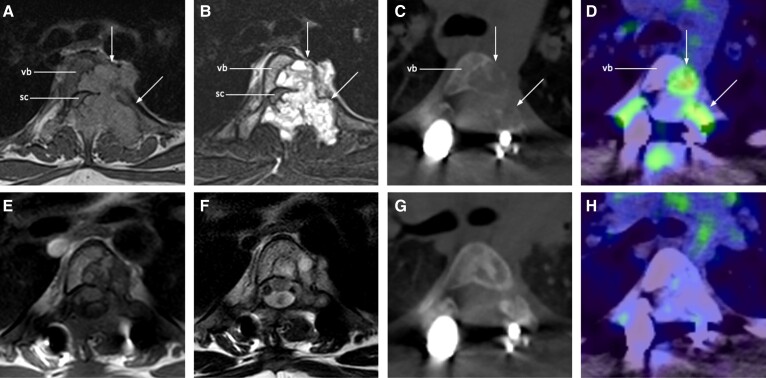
Representative images of denosumab treatment in spinal ABC. MRI T1-weighted (A) and STIR (B) images show a lobulated, expansile lesion (arrows) of T4 vertebral body (vb) and posterior elements with spinal cord (sc) stenosis, before partial resection and spinal stabilization with rods and bone graft. Postsurgery but before denosumab, the remaining ABC is seen as a lytic lesion in the left T4 vertebral body and pedicle on low-dose CT (C) with FDG avidity on PET (D). After 6 months of denosumab treatment, MRI T1-weighted (E) and T2-weighted (F) images show reduced signal intensity, noting metallic artifact from spinal rods reduces quality of images; peripheral sclerosis with increased radiodensity is seen on low-dose CT (G) and resolution of FDG-avidity on PET (H). ABC, aneurysmal bone cyst; CT, computed tomography; FDG, fluorodeoxyglucose; MRI, magnetic resonance imaging; PET, positron emission tomography; STIR, short tau inversion recovery.
